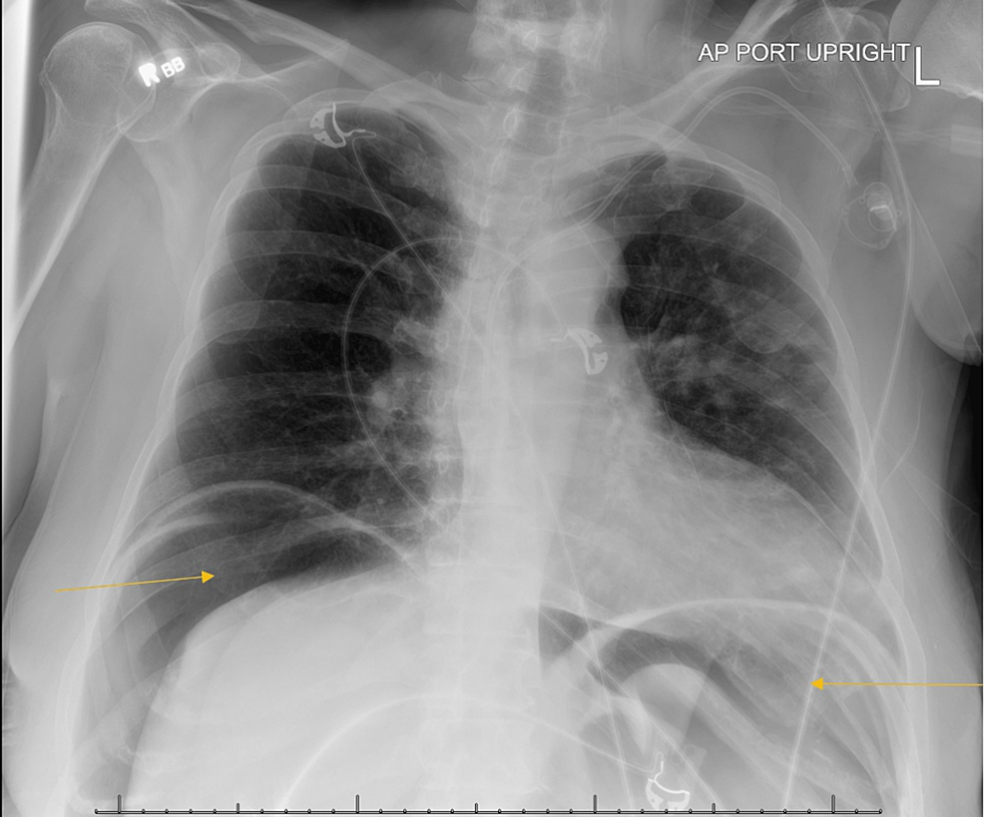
Chest X-ray performed after sigmoidoscopy demonstrating bilateral nodular infiltrates and intra-abdominal air (arrows).
